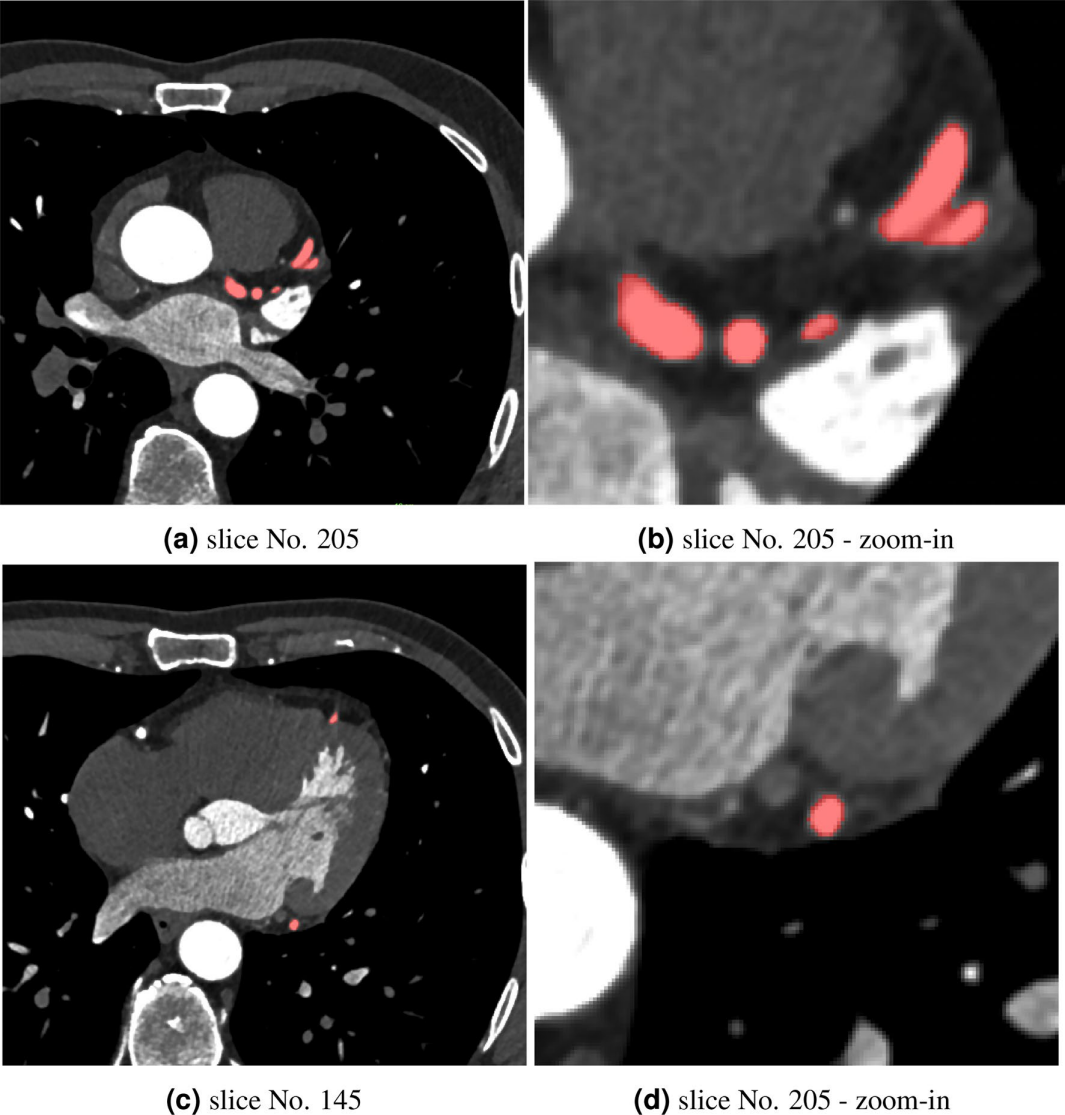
Segmented arteries overlaid on the CT images. Image (a) shows data from slice No. 205. Image (b) presents a zoom-in on the arteries of interest. Image (c) shows data from slice No. 145, and image (d) presents a zoom-in on the arteries of interest.
